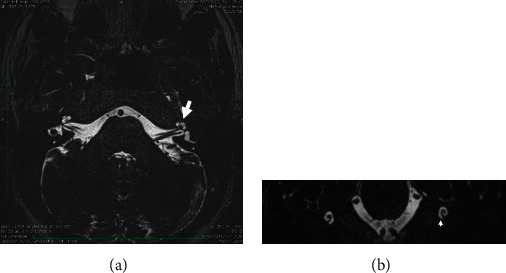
Magnetic resonance imaging of the temporal bone: axial (a) and coronal (b) CISS 3D slices showing a signal of discreetly lower intensity in the left cochlea compared to the right one.
